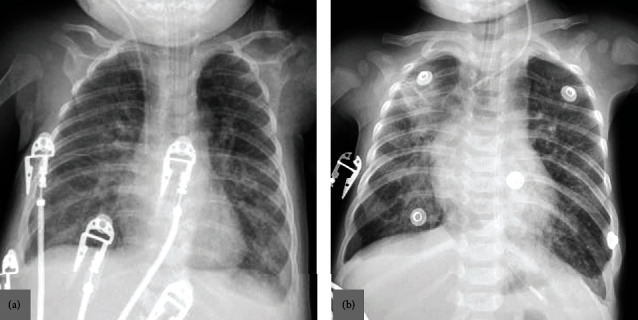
Chest X-rays of two different patients with positive COVID-19 RT-PCR test. (a) A 4-month-old male with a neurometabolic disorder was presented with fever and dyspnea. Chest X-ray shows an ill-defined ground-glass opacity as well as reticular areas of increased opacity involving almost all lung zones on both sides, which is an indeterminate chest radiographic finding of pediatric COVID-19 pneumonia. Also noted are the endotracheal tube and a central line. The patient developed severe hypoxemia, was transferred to the intensive care unit, was mechanically ventilated, and died. (b) A 4-month-old male with Down syndrome was presented with fever and respiratory distress. Chest X-ray shows an ill-defined area of consolidation and ground-glass opacity in the right upper and lower lung zones along with ill-defined diffuse reticular areas of increased opacity in both lungs, which is an indeterminate chest radiographic finding of pediatric COVID-19 pneumonia. Also noted are the endotracheal tube and a central line The patient developed progressive respiratory compromise and was transferred to the intensive care unit, was mechanically ventilated, and died.
